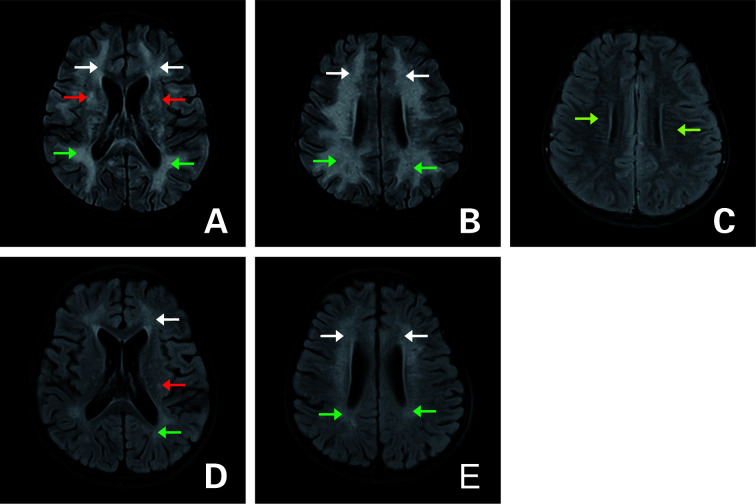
Axial fluid attenuation inversion recovery (FLAIR) MRI of the brain (A–C) upon admission and (D, E) 3 months later. (A, B) T2-hyperintense lesions in the white matter of bilateral cerebral hemispheres (white arrow: frontal lobe; green arrow: parietal lobe), bilateral basal ganglia (red arrow). (C) Periventricular radial linear enhancement (light green arrow). (D, E) Follow-up images of improved T2 lesions.
